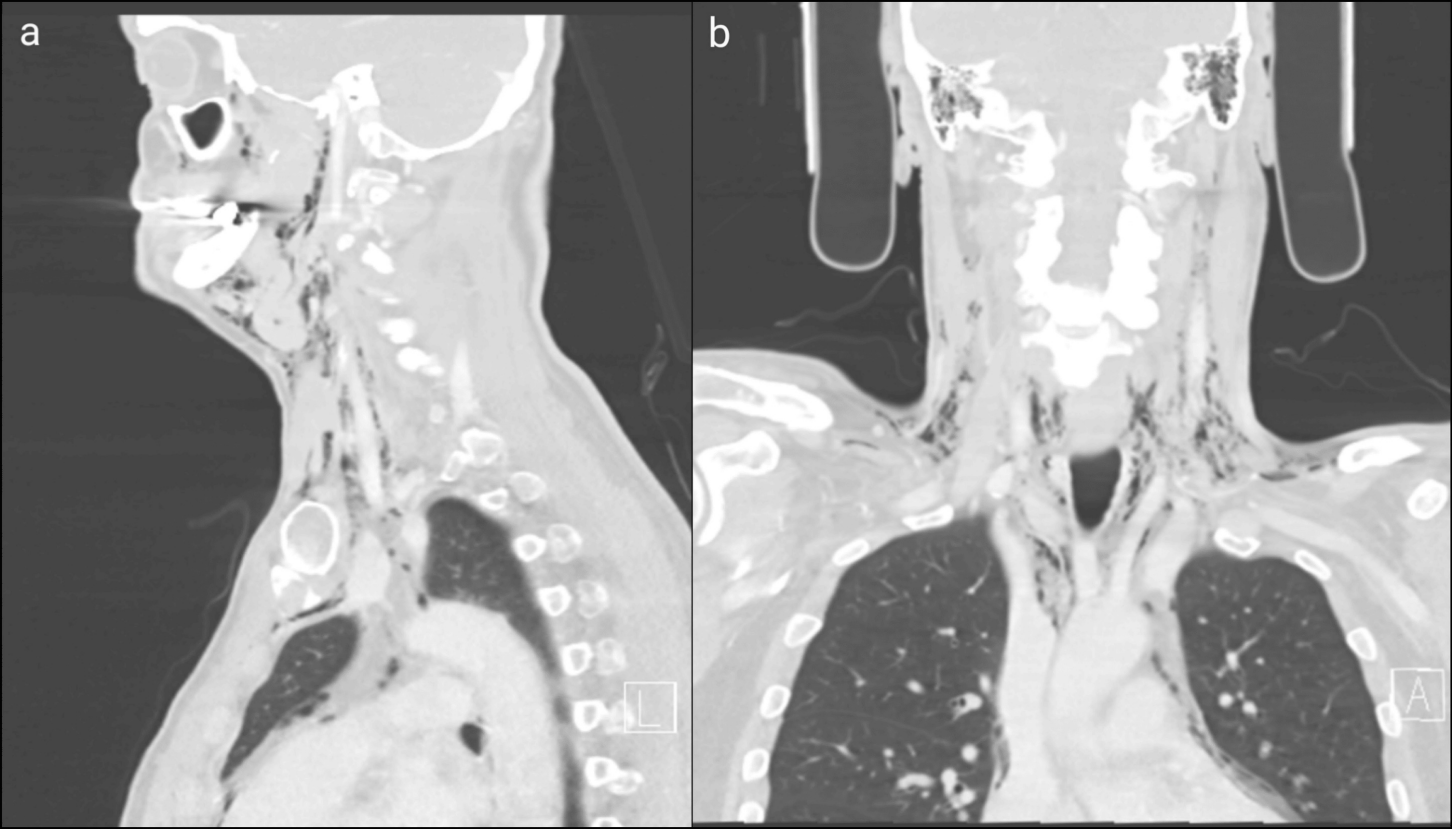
Multiple CT scan views showing extended emphysema: (a) sagittal plane and (b) coronal plane CT: computed tomography
